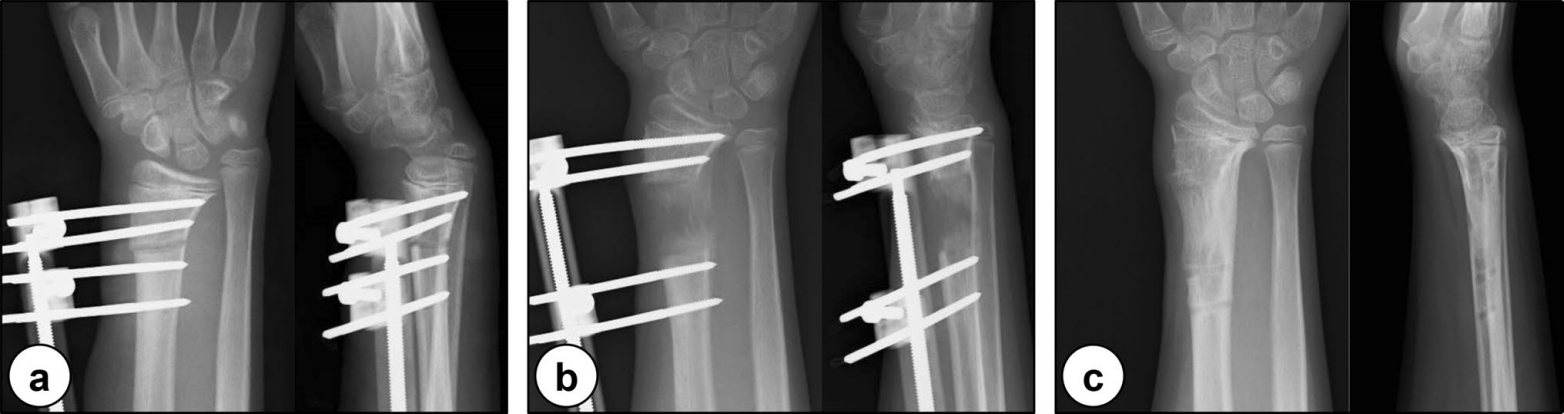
Anteroposterior (left) and lateral (right) radiographs during the gradual lengthening with distraction osteogenesis: a at the start of lengthening 1 week postoperatively; b at the end of lengthening, 7 weeks postoperatively; and c at the adequate maturation of the callus at the bone interval, 18 weeks postoperatively
